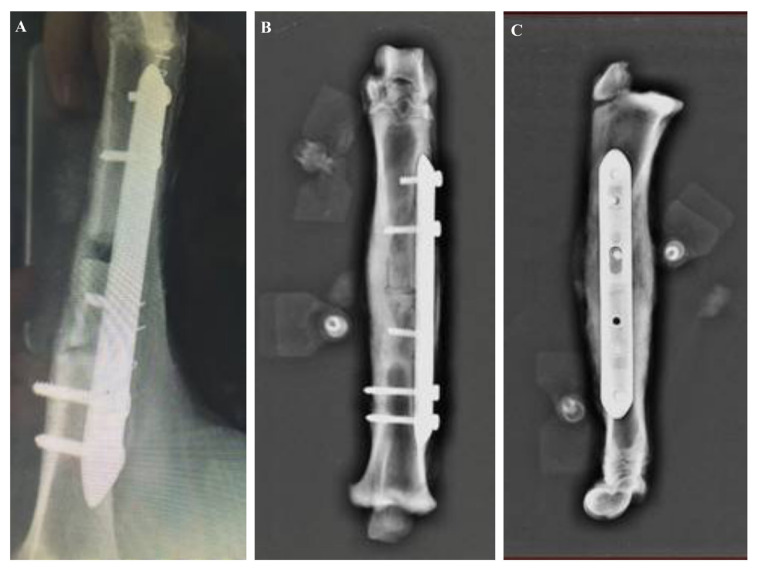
Radiographic evaluation of the surgical site at (a) 4 weeks and (b,c) 12 weeks.
